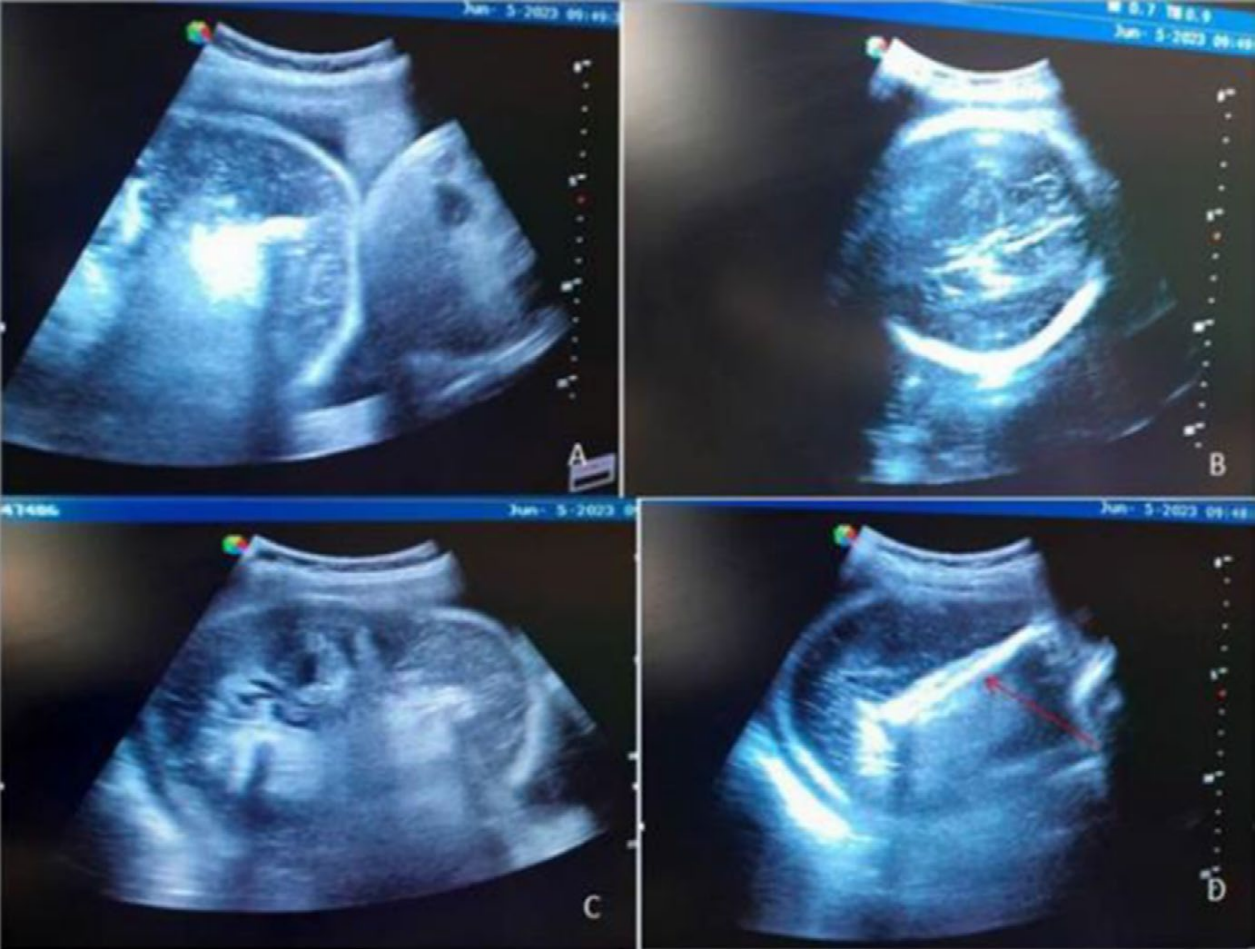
Ultrasound of features of TRAPS. (A) Pump twin at the left side of the uterine cavity and acardiac twin at the right upper side of the uterine cavity. (B) Head of pump twin. (C) The acardiac fetus—large cystic‐like tissue without visualization of cardiac activity, head, or bilateral upper limbs. (D) Femur bone of acardiac twin (red arrow).
